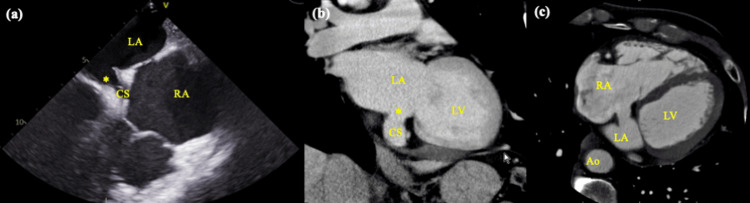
Preoperative findings. (a) Transesophageal echocardiography showing a defect along the roof of the coronary sinus, creating a direct communication between the left and right atria, consistent with coronary sinus-type atrial septal defect (CS-ASD). (b, c) Contrast-enhanced CT imaging showing a discontinuity in the wall of the coronary sinus, indicating the presence of a septal defect opening into the CS, as well as a right aortic arch. Ao, aorta; CS, coronary sinus; LA, left atrium; LV, left ventricle; RA, right atrium *Unroofed portion of the coronary sinus
